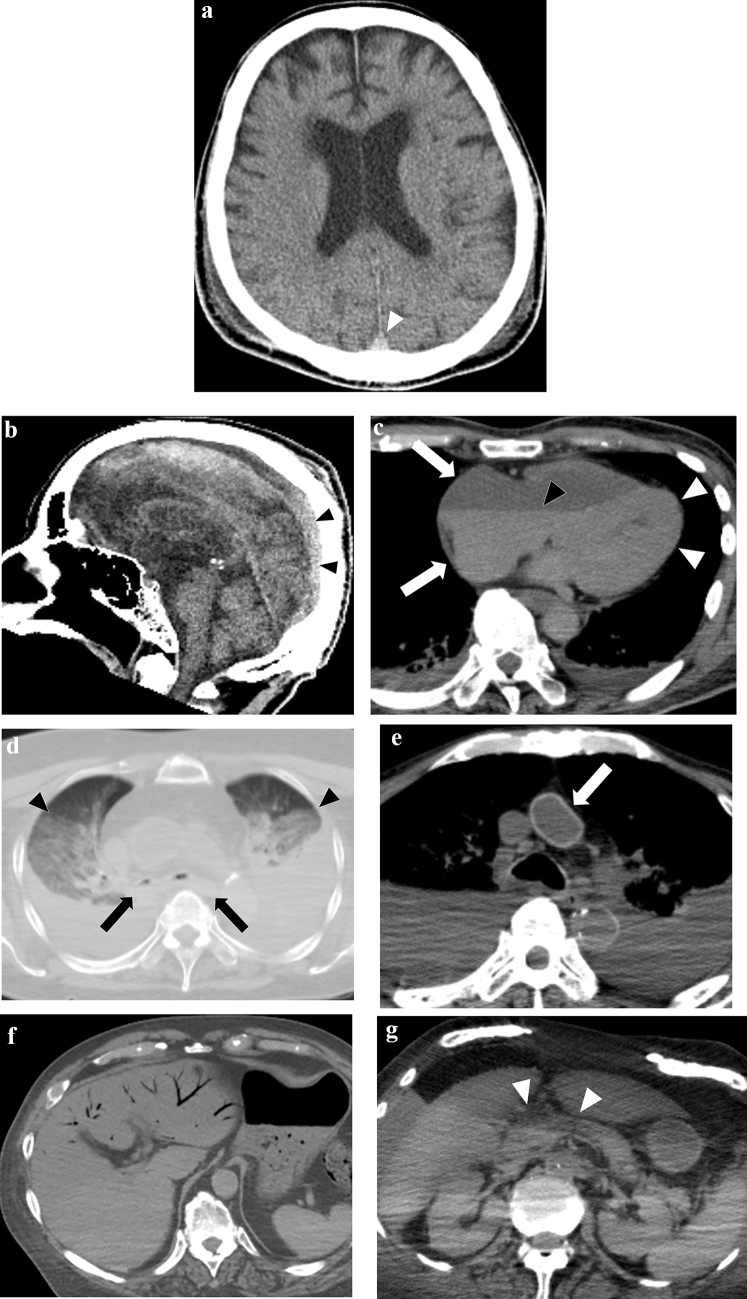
Representative CT images of early regular postmortem changes. a, b increased density in the superior sagittal sinus (arrowheads), brain edema (dorsally dominant), and loss of the differentiation between the gray and white matter. c thickened left ventricular wall (white arrowheads), hyperattenuating in the gravity side (black arrowhead), and dilatation in the right heart cavities (arrows). d fluid in the bilateral main bronchus (arrows) and the increased attenuation, such as ground-glass opacities with the horizontal plane formation on the gravity-dependent side of the lungs (arrowheads). e narrowed short diameter of the aorta and the hyperattenuating and thickened aortic wall (arrow). f intrahepatic portal venous gas (8 h elapsed from death without cardiopulmonary resuscitation). g obscuring of outline and swelling in the pancreas (arrowheads)
